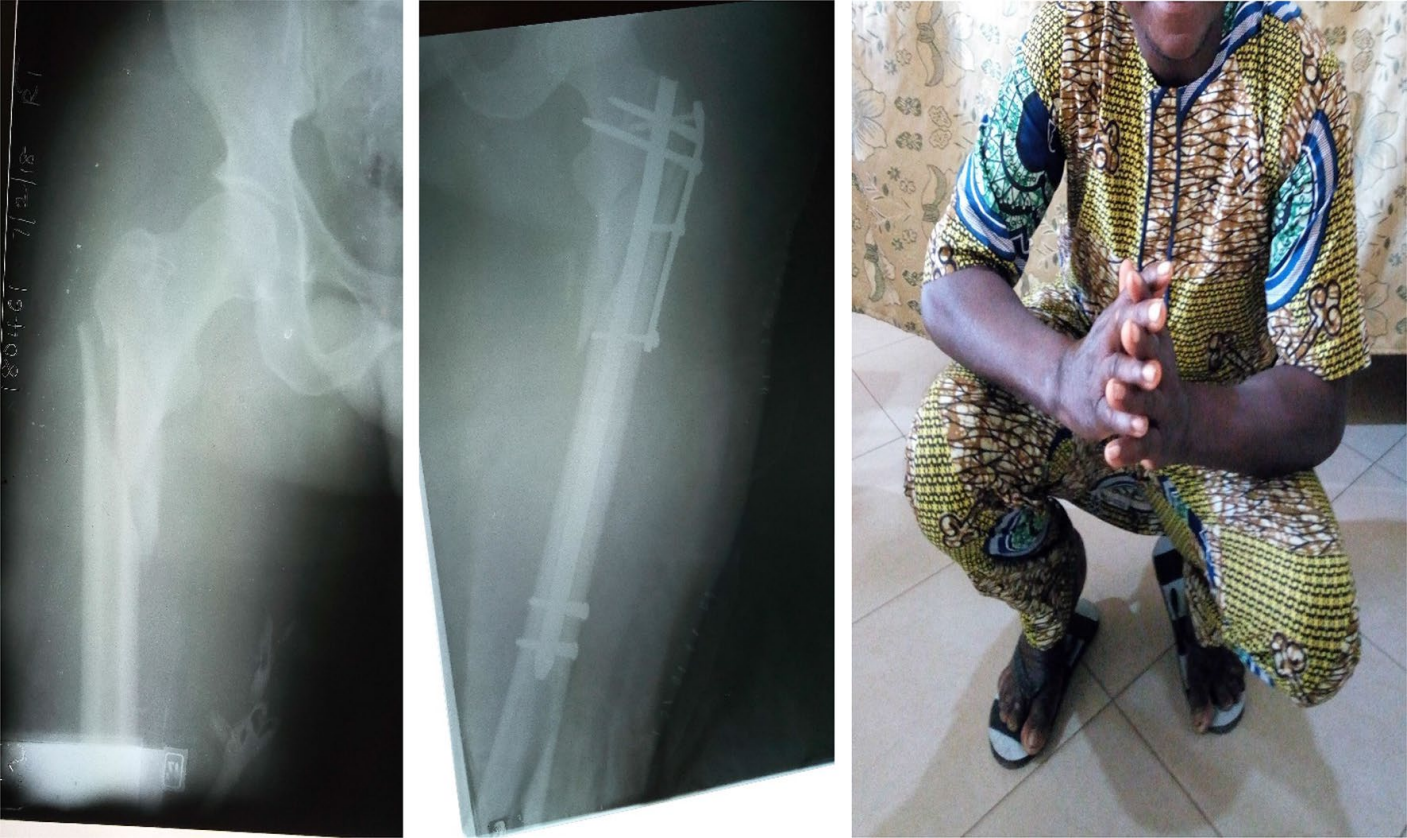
Pre- and post-operative radiographs and “squat and smile” photograph (6-week follow-up) of a 33-year old man who had the SIGN nail combined with the SIGN HV plate to treat a reverse oblique femur fracture with diaphyseal extension.
